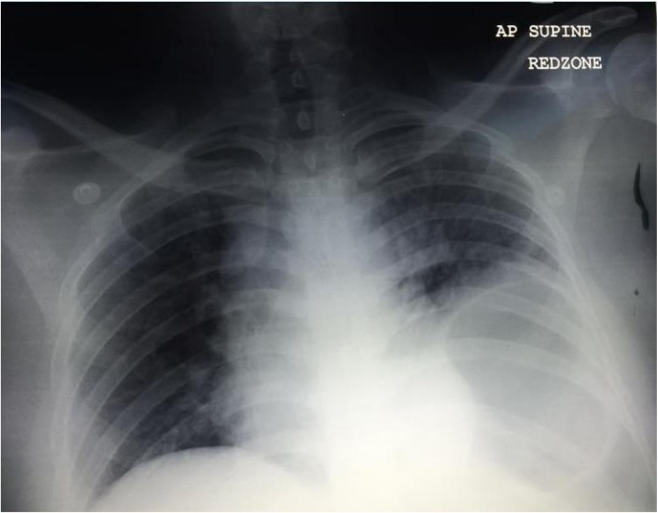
Chest x-ray showing a raised left hemidiaphragm with the presence of stomach shadow in the lower half of the left hemithorax
